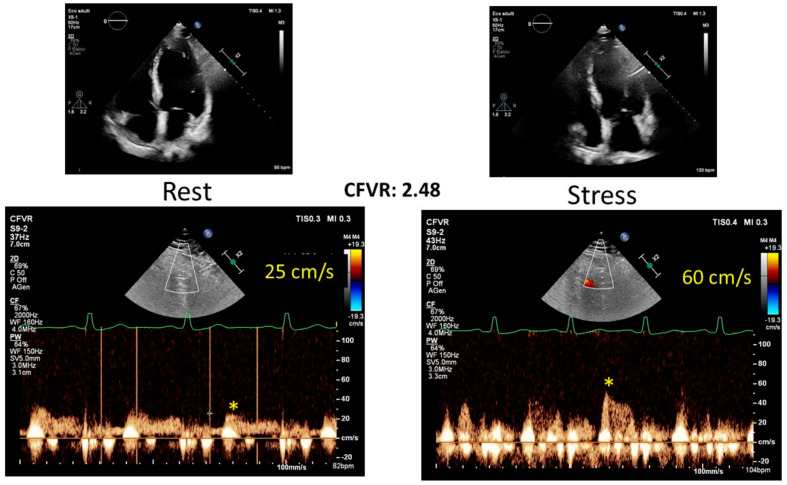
Exercise stress echocardiography in an endurance athlete; the global and regional left ventricular function was within normal limits at rest and during effort, with a normal increase in coronary flow and normal coronary flow reserve (>2). CFVR: coronary flow vascular reserve.
